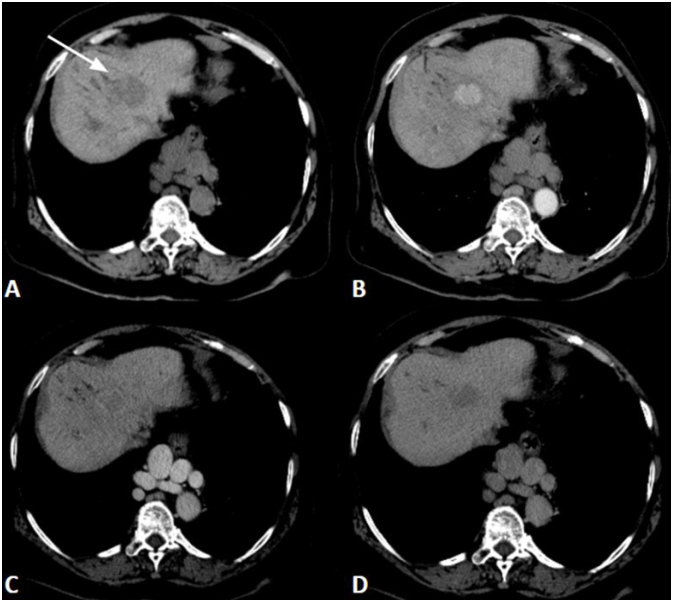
Hepatocellular carcinoma in a 68-year-old woman with hepatosplenic schistosomiasis. A: Unenhanced axial computed tomography image showing hypoattenuating nodule (arrow); B: Hypervascularized nodule in arterial phase; C and D: Washout in delayed phase.
